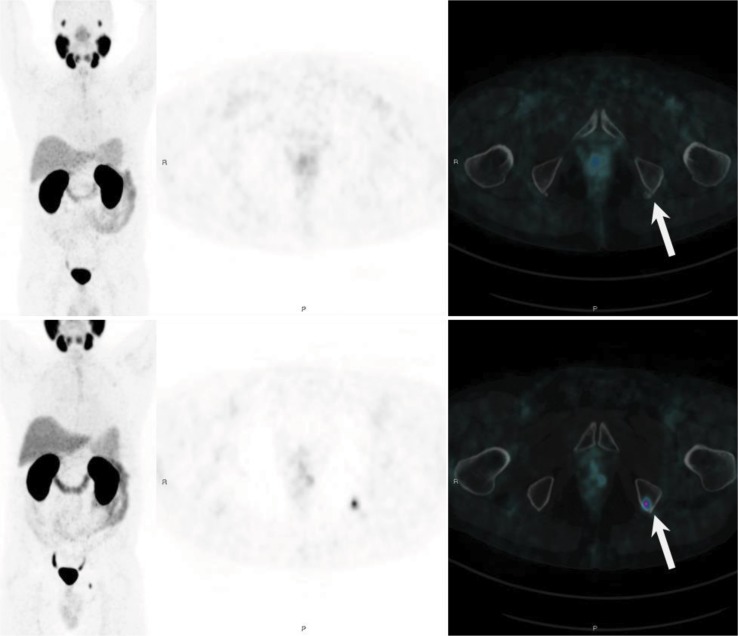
PSMA PET showing long-term PSMA expression against ADT in a patient with recurrent PCa who previously underwent pelvic radiation. The bottom row shows a bone metastatic lesion in left ischiatic tuberosity. The top row shows a new PSMA PET scan performed 10 months after beginning ADT, with resolution of the molecular expression of PSMA, probably reflecting a therapeutic effect of ADT.
